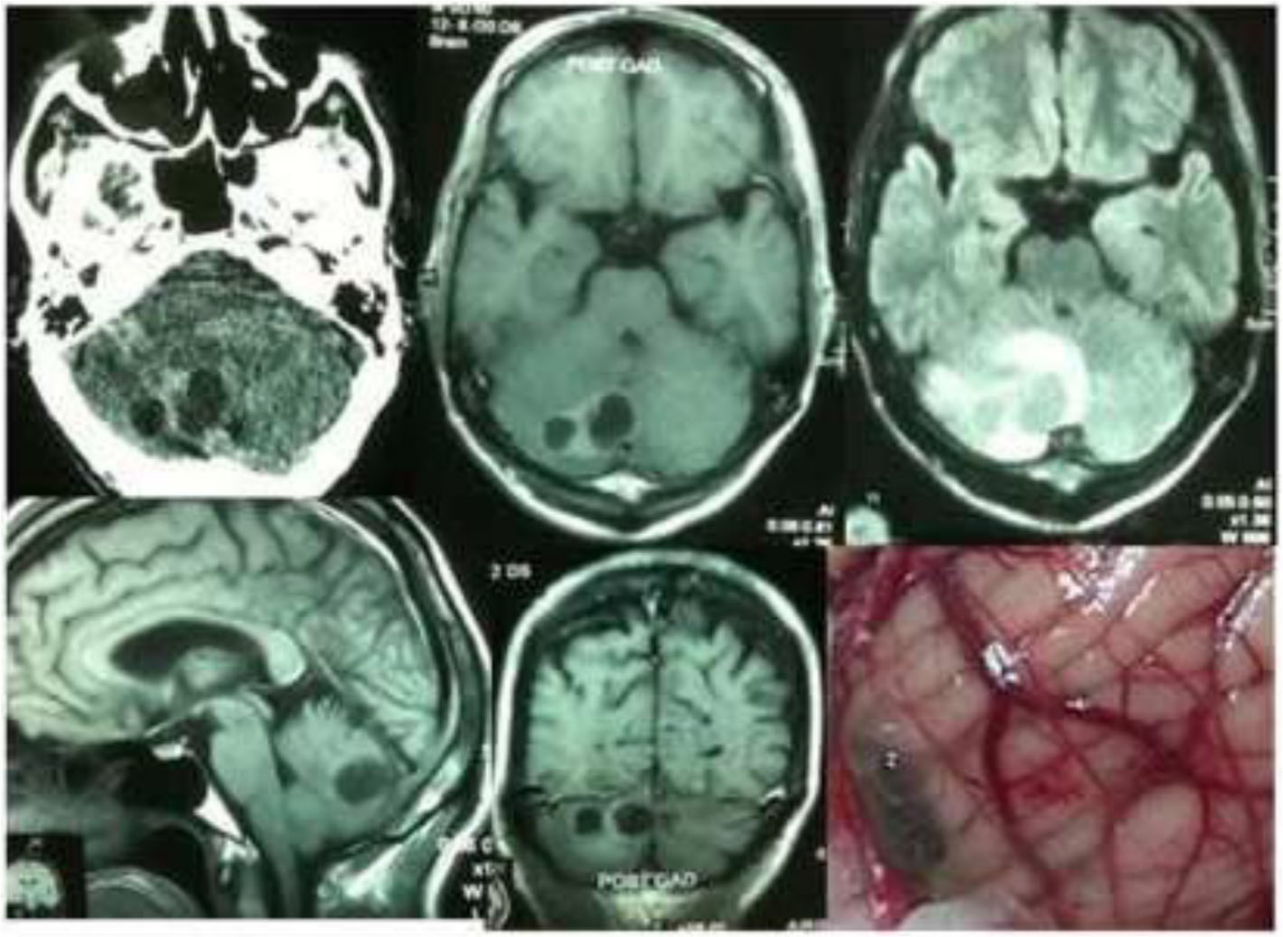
Metastatic lesion in right cerebellar lobe of a 62-year-old female revealed on CT, MR, and intra-operative images
